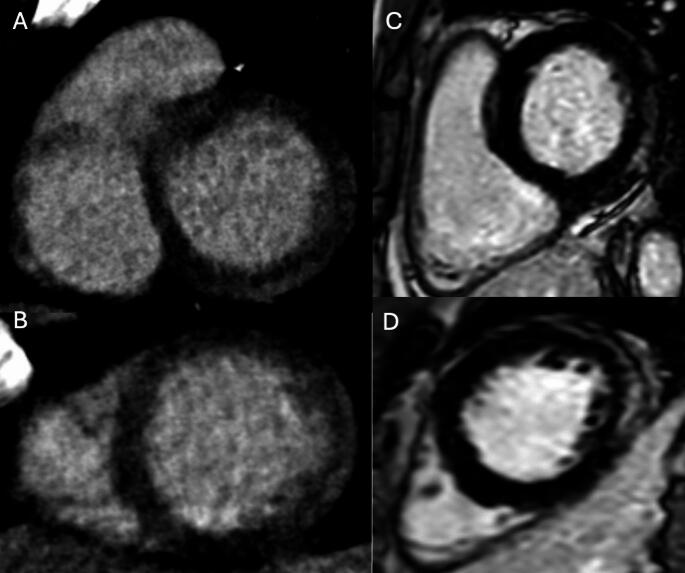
Comparison of late iodine enhancement (LIE) on cardiac CT and late gadolinium enhancement (LGE) on cardiac magnetic resonance (CMR) in a patient with acute myocarditis. Basal and mid-ventricular short-axis CT images (Panels A and B) show subepicardial late iodine enhancement in the anterolateral and inferolateral walls. Corresponding CMR images in the same short-axis views (C and D) confirm the presence and distribution of LGE in the same myocardial segments
